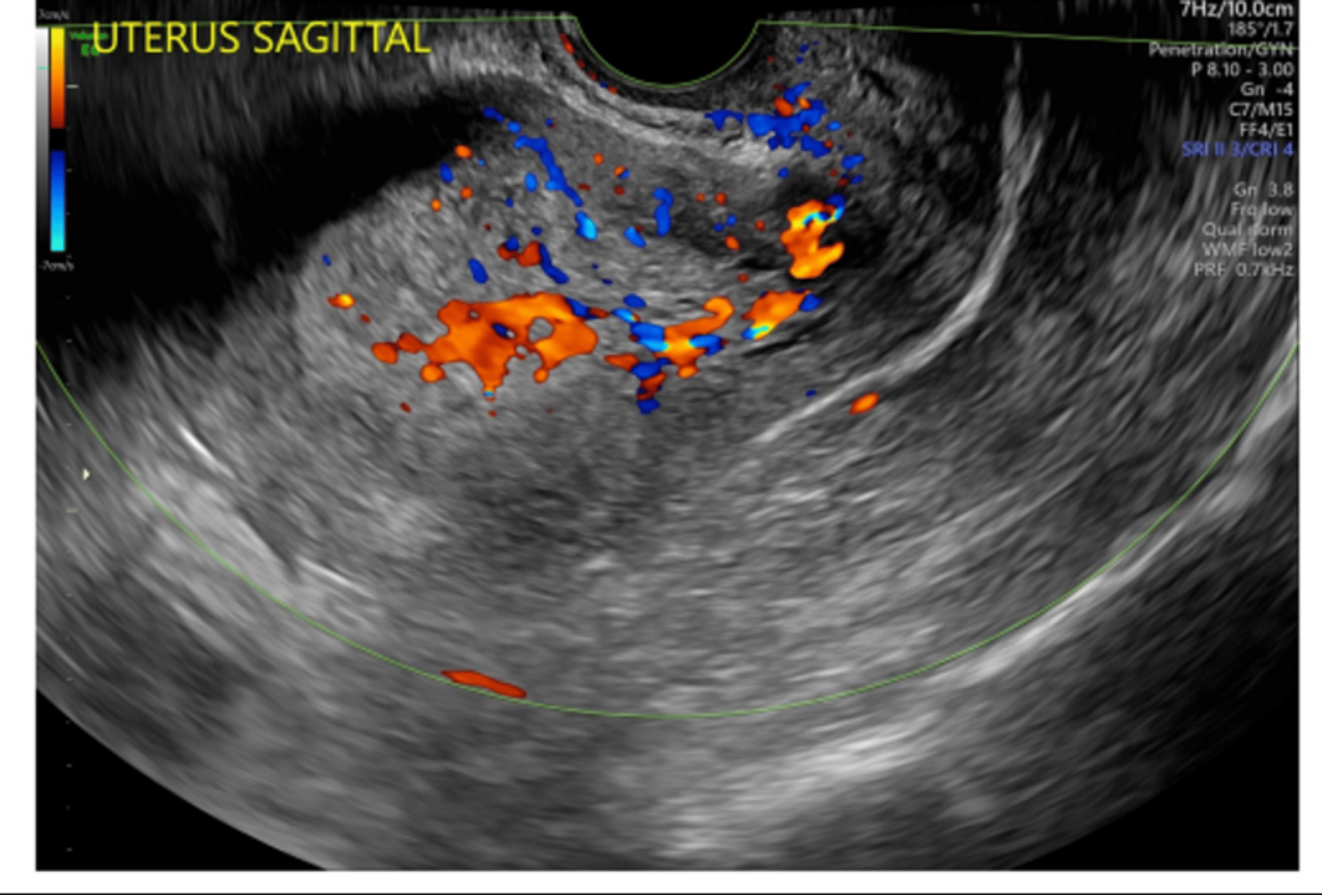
Ultrasound of the uterus, in the sagittal view, showing increased vascularity of the myometrium, suggestive of adenomyosis.
